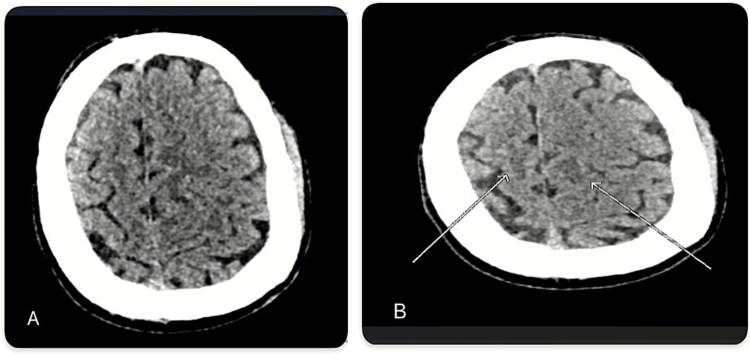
(A and B): Non-contrast computed tomography (CT) imaging demonstrates hypodensity in the left high parasagittal cortex and its underlying white matter, accompanied by faint hypodensity in the right white matter.
